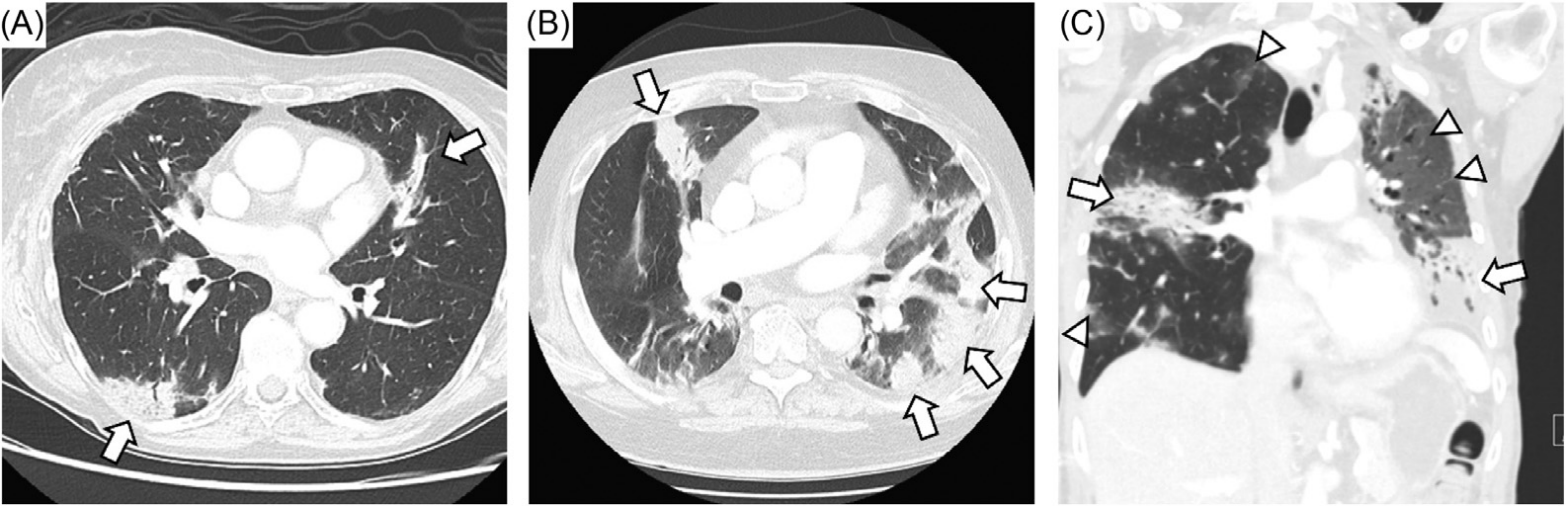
Representative case of immune checkpoint inhibitor- (ICI) associated pneumonitis. A) Elderly woman with melanoma treated with ICI presented with patchy bilateral consolidative opacities without any respiratory symptoms (Grade 1). B) Elderly man with melanoma affecting scalp underwent surgery followed by neoadjuvant ICI presented with persistent dry cough. Computed tomography of the chest (CT chest) revealed multifocal consolidative opacities. He underwent bronchoscopy with biopsy of lymph nodes and bronchoalveolar lavage without evidence of malignancy or infection. He was diagnosed with Grade 2 ICI-associated pneumonitis, and he improved with oral steroids. C) Middle-aged woman with triple negative breast cancer on ICI presented with cough and dyspnea with exertion not improved on outpatient oral steroid therapy. On physical exam she was noted to be tachypneic and hypoxic on room air. Coronal CT chest revealed consolidative opacities on the right and left along with ground-glass infiltrates on the left upper lobe. She was admitted and treated for Grade 3 ICI-associated pneumonitis with intravenous methylprednisolone (1 mg/kg) followed by infliximab. She improved and was discharged on prolonged steroid taper.
